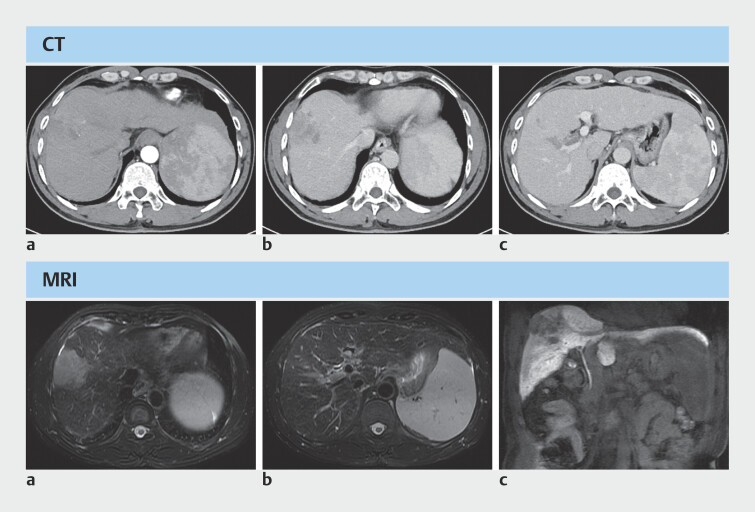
Enhanced computed tomography (CT) and magnetic resonance imaging (MRI) at the patient’s first admission. CT images:
